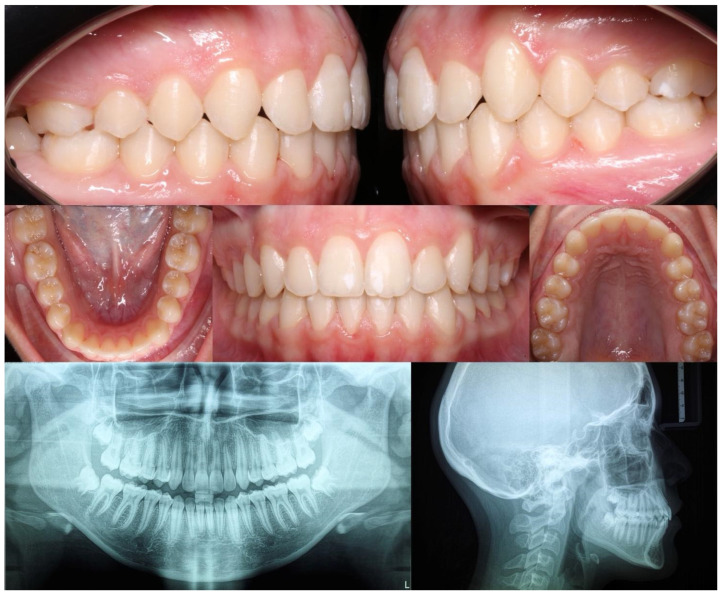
Final right, left, front, upper, and lower orthopantomography and lateral cephalometric radiographs.
